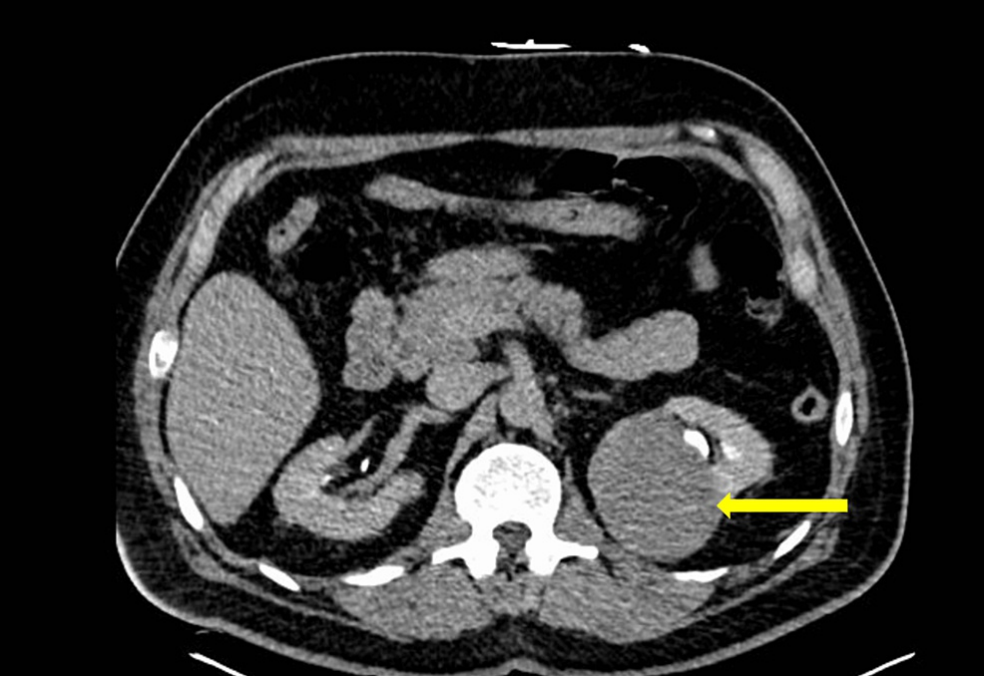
CT urogram with a large left renal mass (yellow arrow) invading the collecting system
